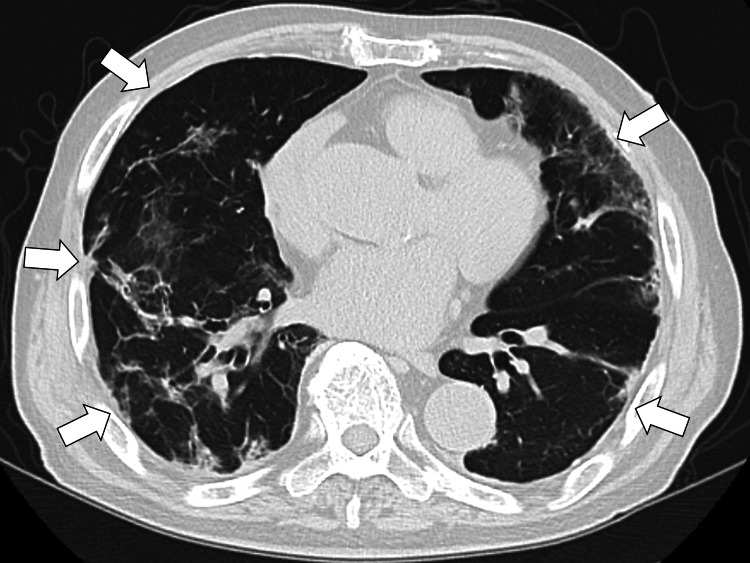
Chest CT one month after discharge showing improvement of the infiltration of both lungs (white arrows) CT, computed tomography
